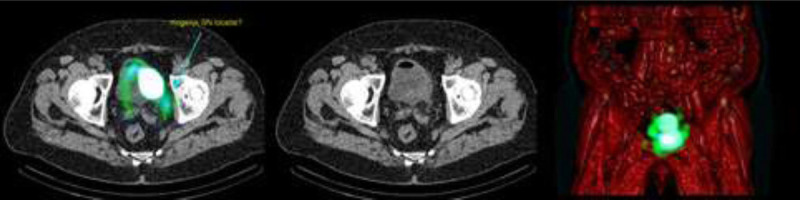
Upper row: Fused SPECT/CT, CT, and volume-rendered fusion image SPECT. Fused images show a focal radioactive signal in the right obturator foramen, marked as the SN. The green arrow on CT shows the corresponding nonenlarged lymph node. Lower row: left: Intraoperative view with white light, right: view of the fluorescent camera. White arrow shows focal ICG uptake and the corresponding lymph node, the SN.
